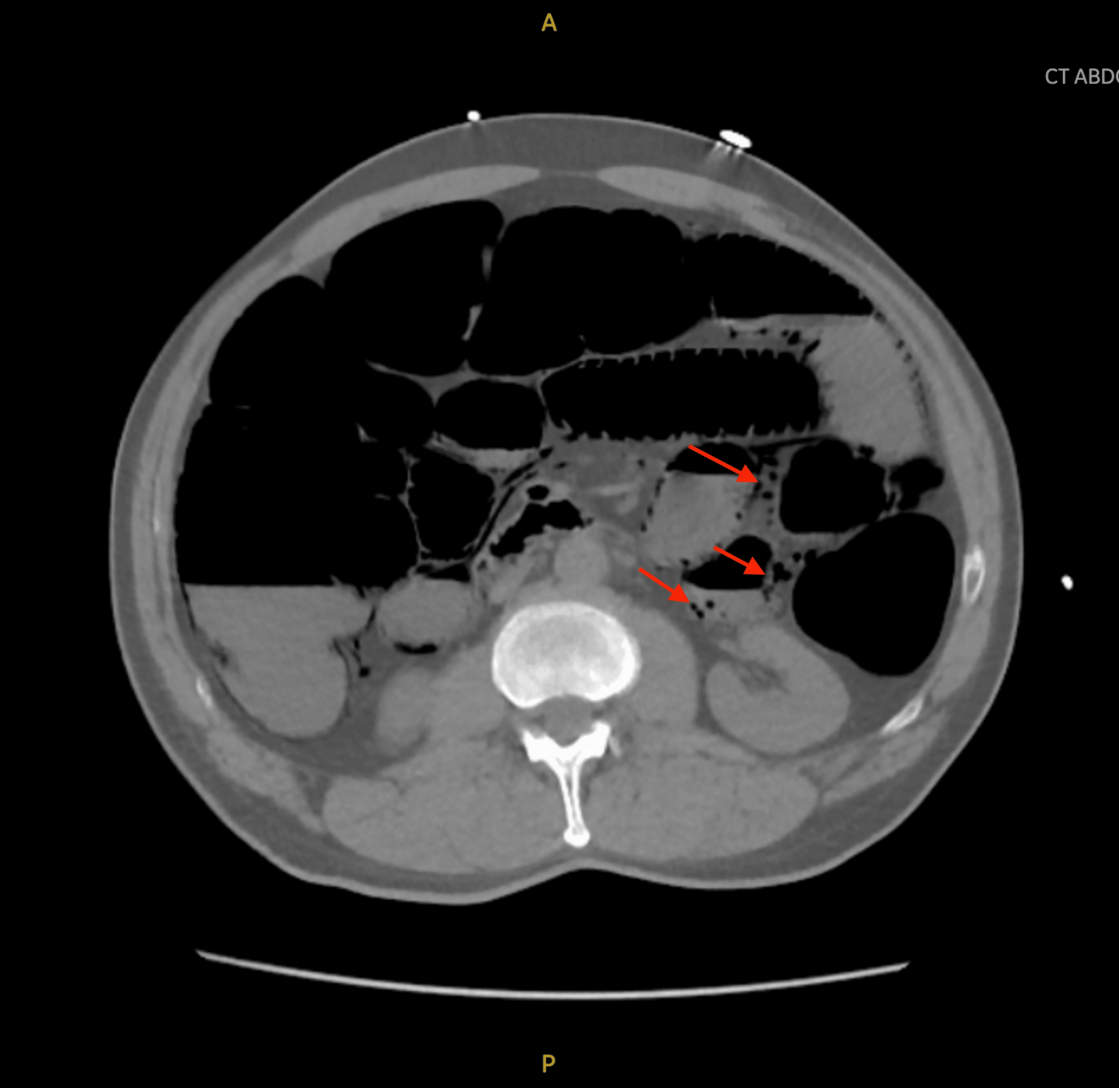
Axial CT scan of the abdomen without contrast demonstrating pneumatosis (red arrows) in the small bowel and diffuse bowel distention
